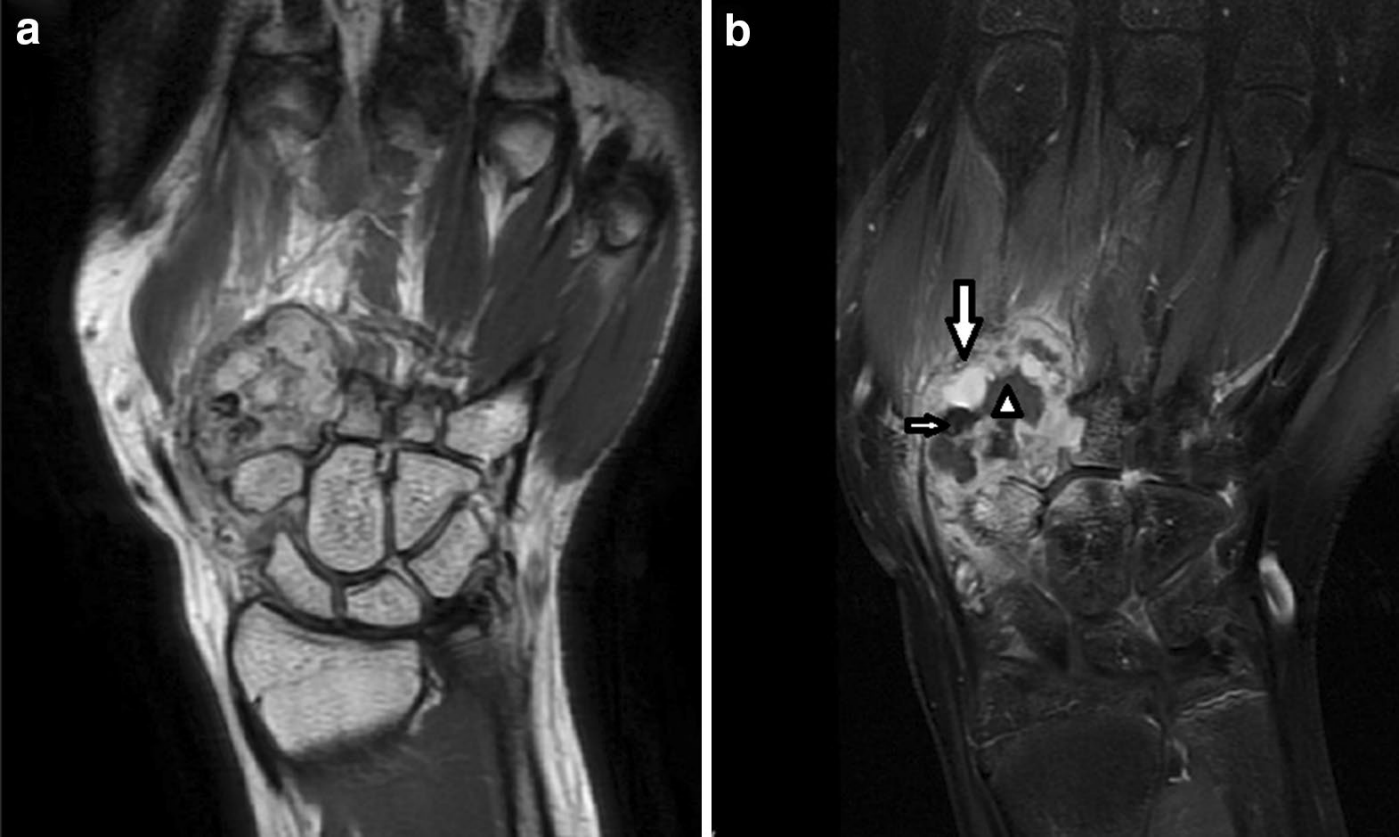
Case 3: a Coronal T2-weighted MR image shows a relatively well-defined mass with heterogeneous hyperintensity on T2WI. b Coronal fat-supressed T1-weighted postcontrast image shows cystic/necrotic components and thrombus (*short arrow*) inside the lesion with very strong enhancement (*long arrow*) of tumor tissue within the lesion and enhancement in the surrounding soft tissue
